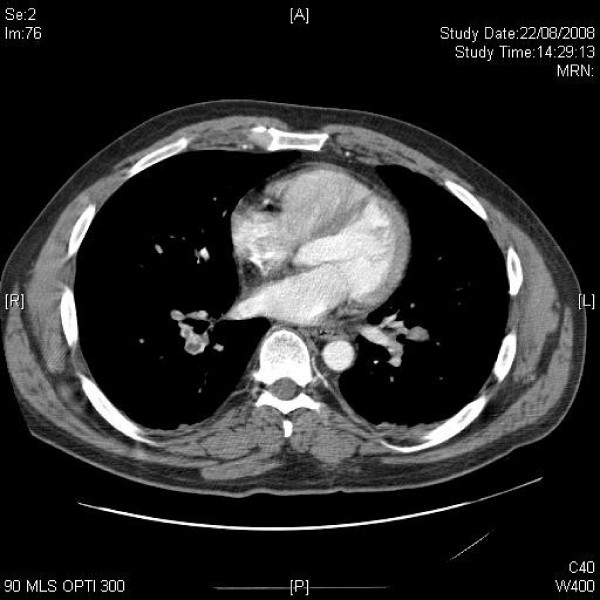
Axial CT of chest shows embolus in the right lower lobar artery.
